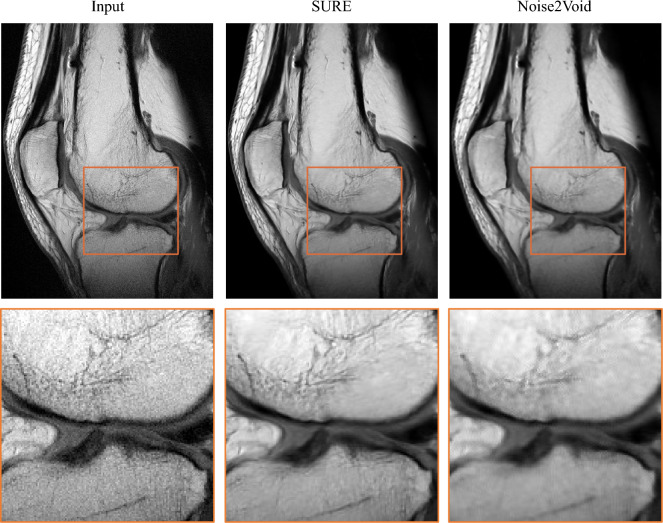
Evaluation of model robustness. The network, trained on T2-weighted 0.55 T head scans, is tested on a PD-wighted knee scan acquired at 1.5 T. The results demonstrate the adaptability of our SURE-based model in reducing image noise while preserving detailed structures and edges. In contrast, the model trained using Noise2Void predicts images with blurred fine structures and details.
